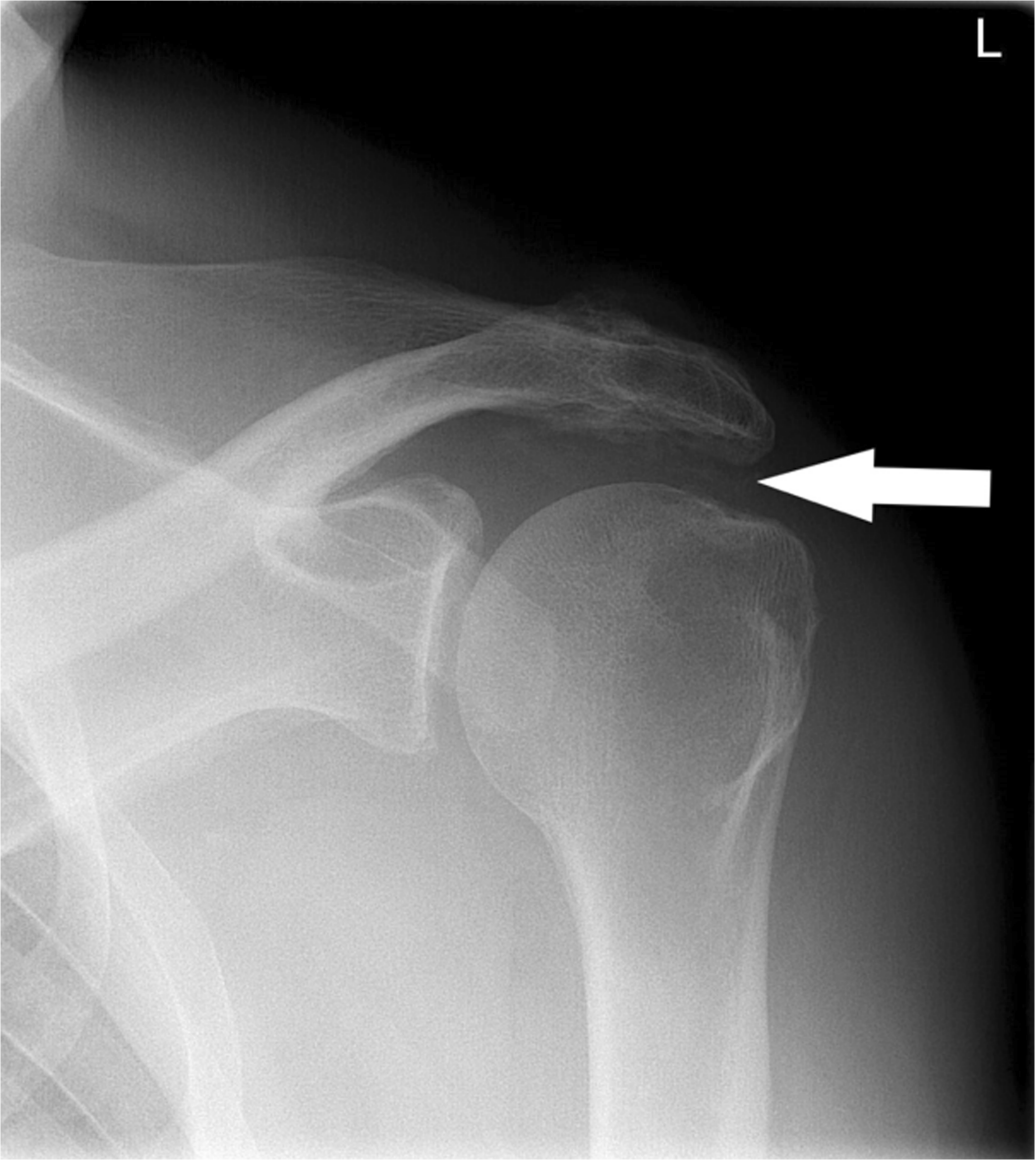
Anteroposterior (AP) conventional radiographs of the shoulder demonstrating several markers. The left shoulder demonstrates calcifications of type C: Non-homogenous calcifications with serrated or irregular contours in the subacromial space are observed
